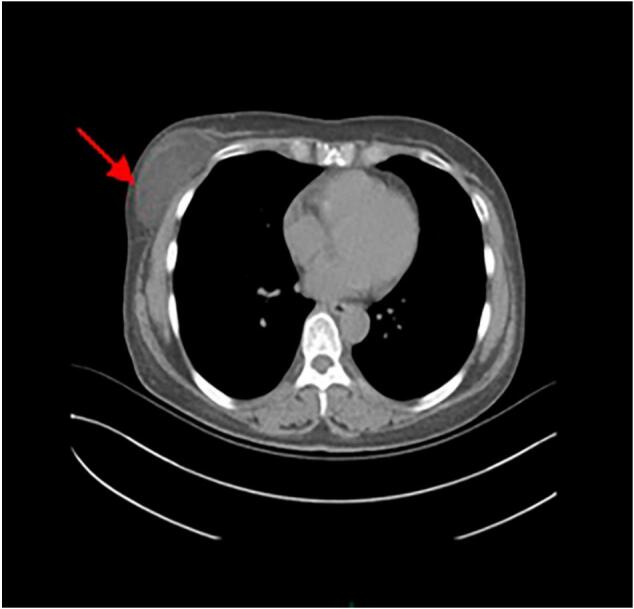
Computed tomography (CT) of the chest showed a heterogeneous low-density shadow (red arrow). (For interpretation of the references to colour in this figure legend, the reader is referred to the web version of this article.)
